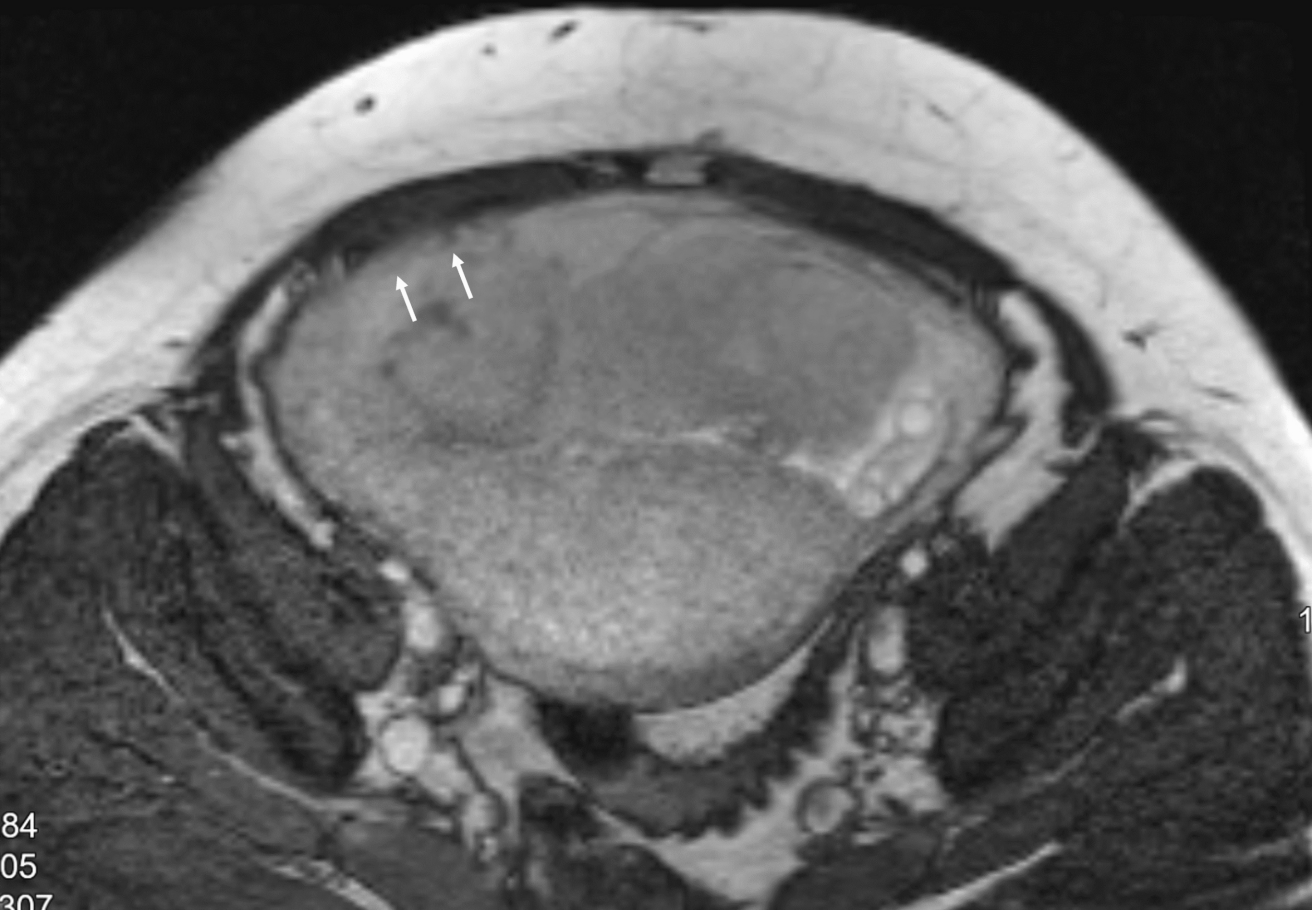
MRI Pelvis Axial view Bridging vessels and increased vascularity suggestive of abnormal placental adherence.
